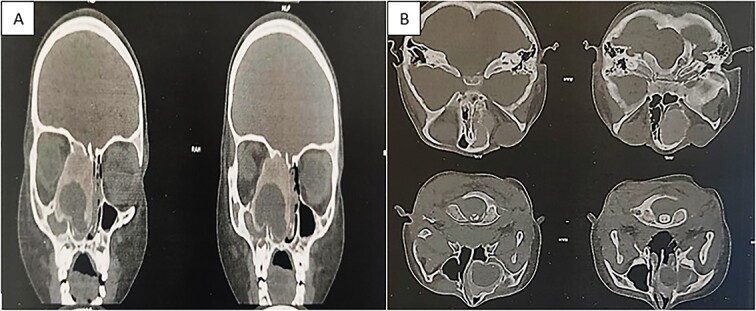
CT showing an expansible, well-demarcated, osteolytic lesion with a peripheral sclerotic rind on the right side of the ethmoid bone, expanding into the ipsilateral nasal cavity, orbit, and maxillary sinus; (A) coronal CT scan; (B) axial CT scan.
